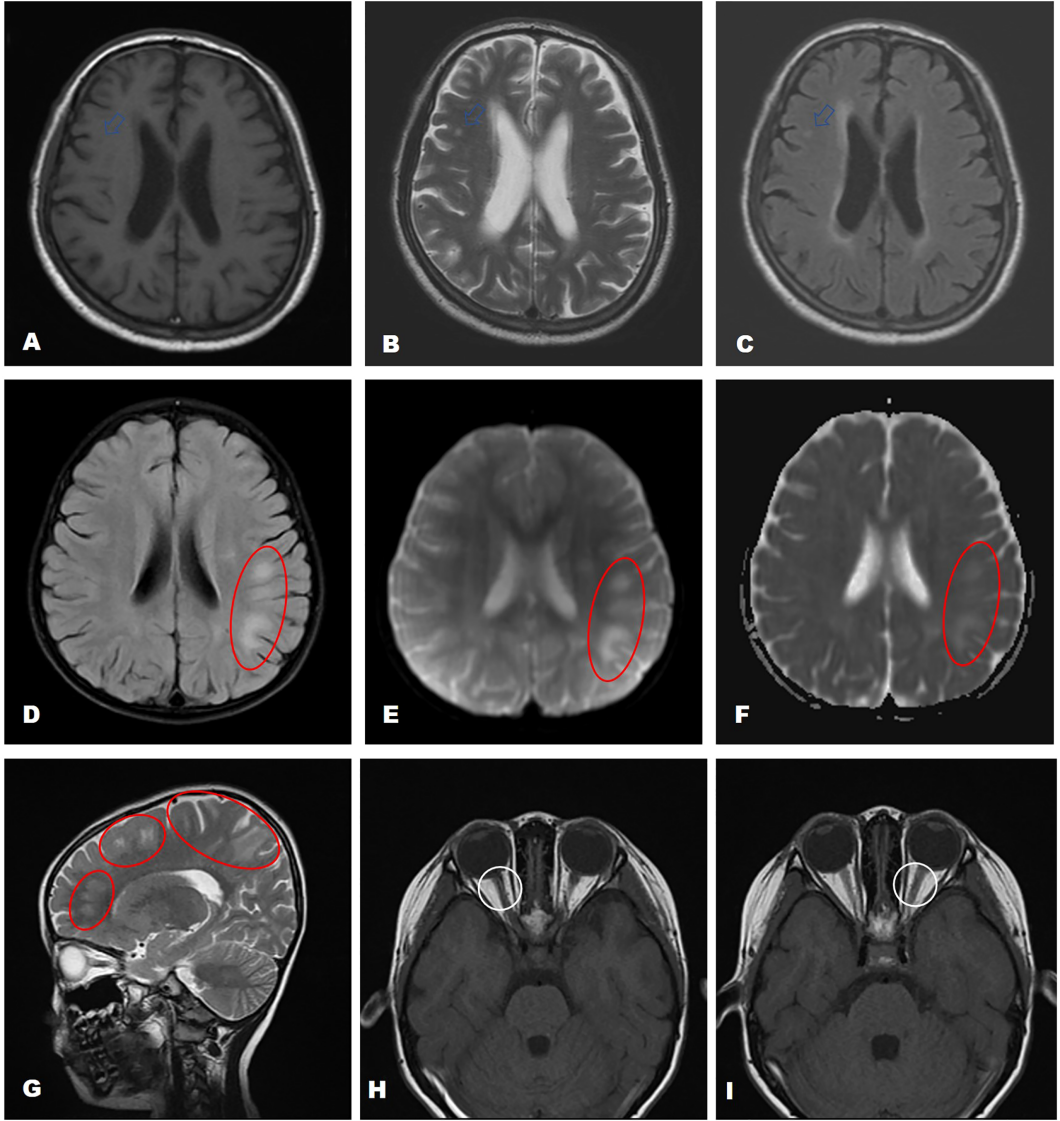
MRI findings of AE patients with coexisting antibodies. The brain MRI of Patient 18 showed a small patchy area with long T1 and long T2 signal shadows in the right frontal lobe with a high T2-FLAIR signal (A–C, marked by a red circle and a white arrow). Patient 19 showed multiple demyelinating lesions in the white matter of the left cerebral hemisphere (D–G, marked by a red circle) and the bilateral optic nerve H, I Fat suppression-T2 sequence).
